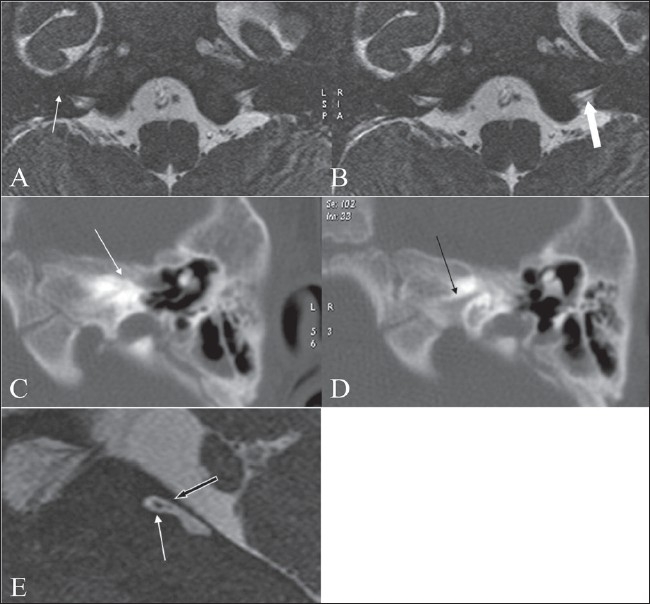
Michel deformity. Axial 3D-FIESTA images (A, B) show absence of the entire vestibulo- cochlear structures bilaterally (arrowd). The internal auditory canals are small on both sides, with a markedly thin eighth nerve (white arrow in B). HRCT images (C, D) shows a small left internal auditory canal (black arrow in D) and absence of the entire vestibulo- cochlear apparatus (white arrow in C), consistent with a Michel deformity. An oblique sagittal 3D FIESTA image (E), through the small internal auditory canal shows a thinned-out eighth nerve (block back arrow) with no divisions and a normal facial nerve antero-superiorly (arrow)
